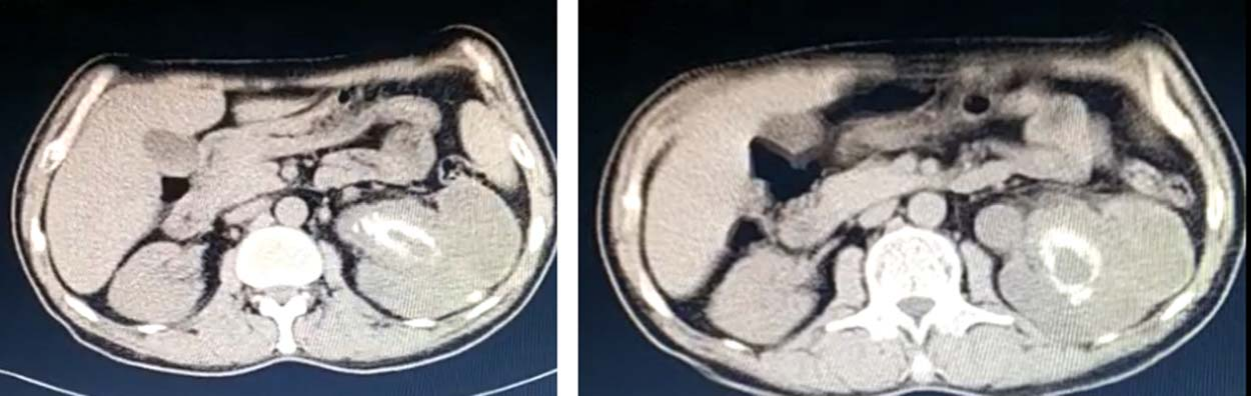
A noncontrast computed tomography axial view of the abdomen showing the enlarged left kidney with cystic pelvicalyceal dilation.
